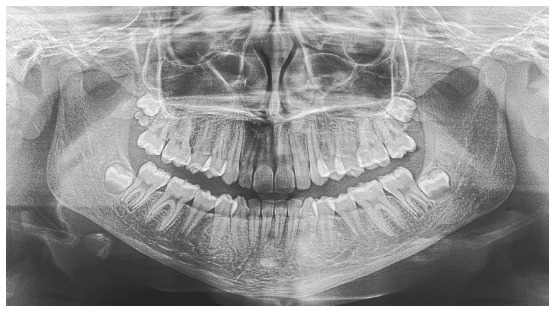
Case 1: Intermediate panoramic radiograph after stage 1.
